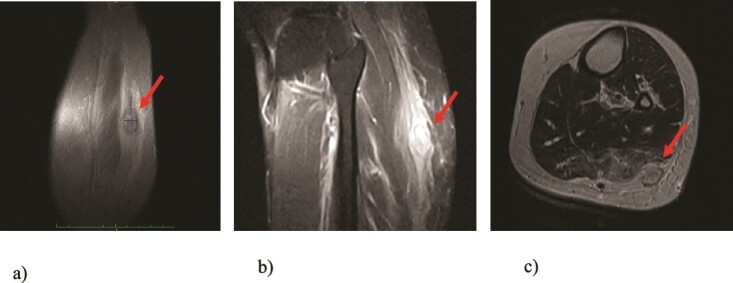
MRI findings of the tumor (arrows): (a) T1 image, coronal view; (b) T2 image, sagittal view; (c) T1 image, axial view revealing presence of a well-defined soft tissue tumor positioned in the subcutaneous fat tissue of the left calf with size of 3 cm and swelling of the surrounding muscles.
